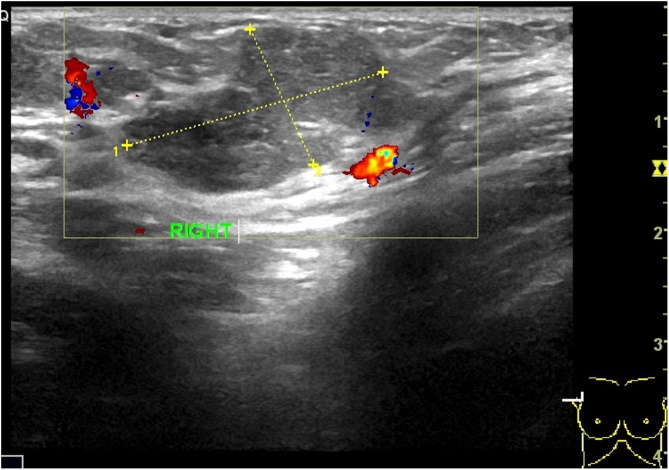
Doppler ultrasonography.
